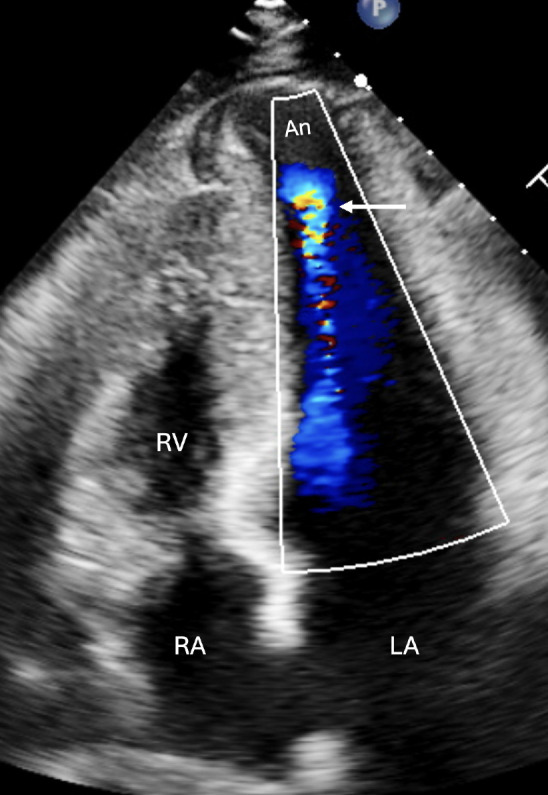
Four-chamber transthoracic echocardiographic view with color Doppler, showing an apical aneurysm (arrow). Abbreviations: An, Aneurysm; LA, Left atrium; RA, Right atrium; RV, Right ventricle.
